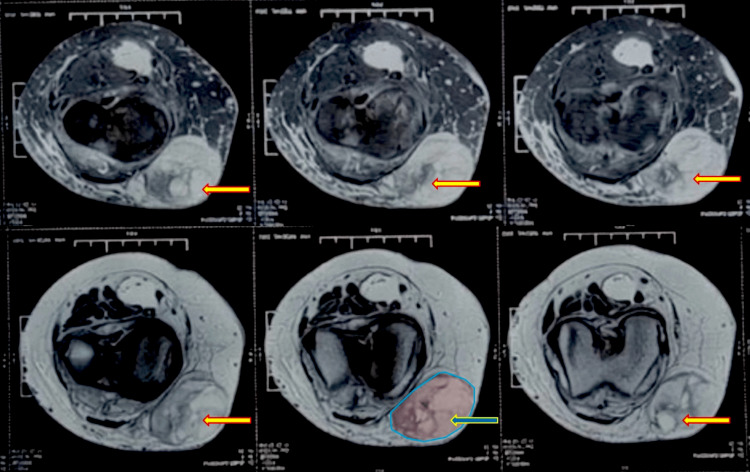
MRI showing a subcutaneous swelling in the anteromedial left knee, closely abutting the underlying bone The yellow arrows indicate the lesion in all the MRI frames and the blue arrow indicates the highlighted lesion adjacent to the knee joint in one frame. MRI, magnetic resonance imaging.
